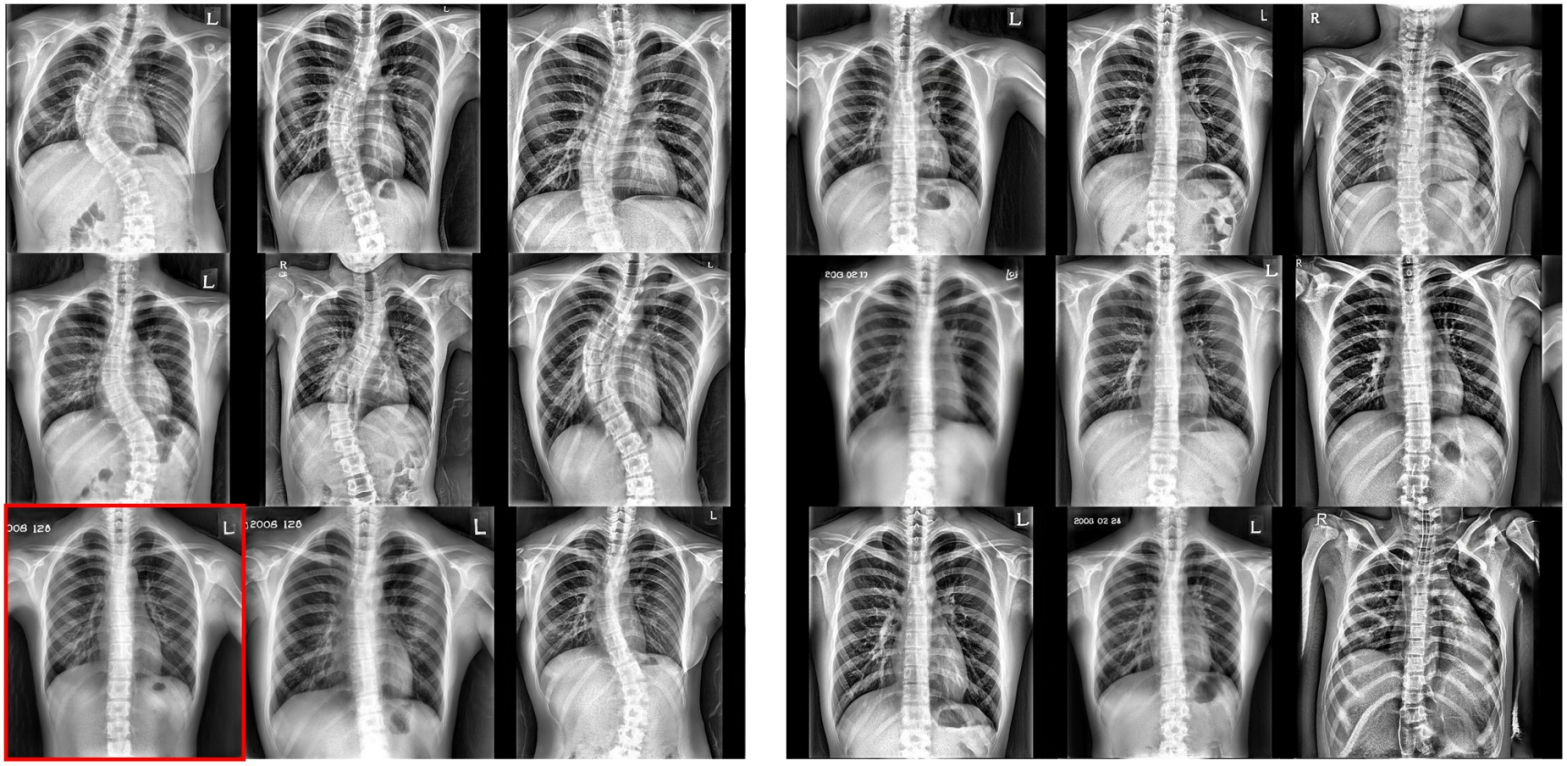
Generated CXR samples. (Left) Samples from a model trained on an imbalanced dataset (our method). (Right) Samples from a model trained on a balanced dataset. Red box: a sample radiograph that can be diagnosed as a normal spine in generated samples.
